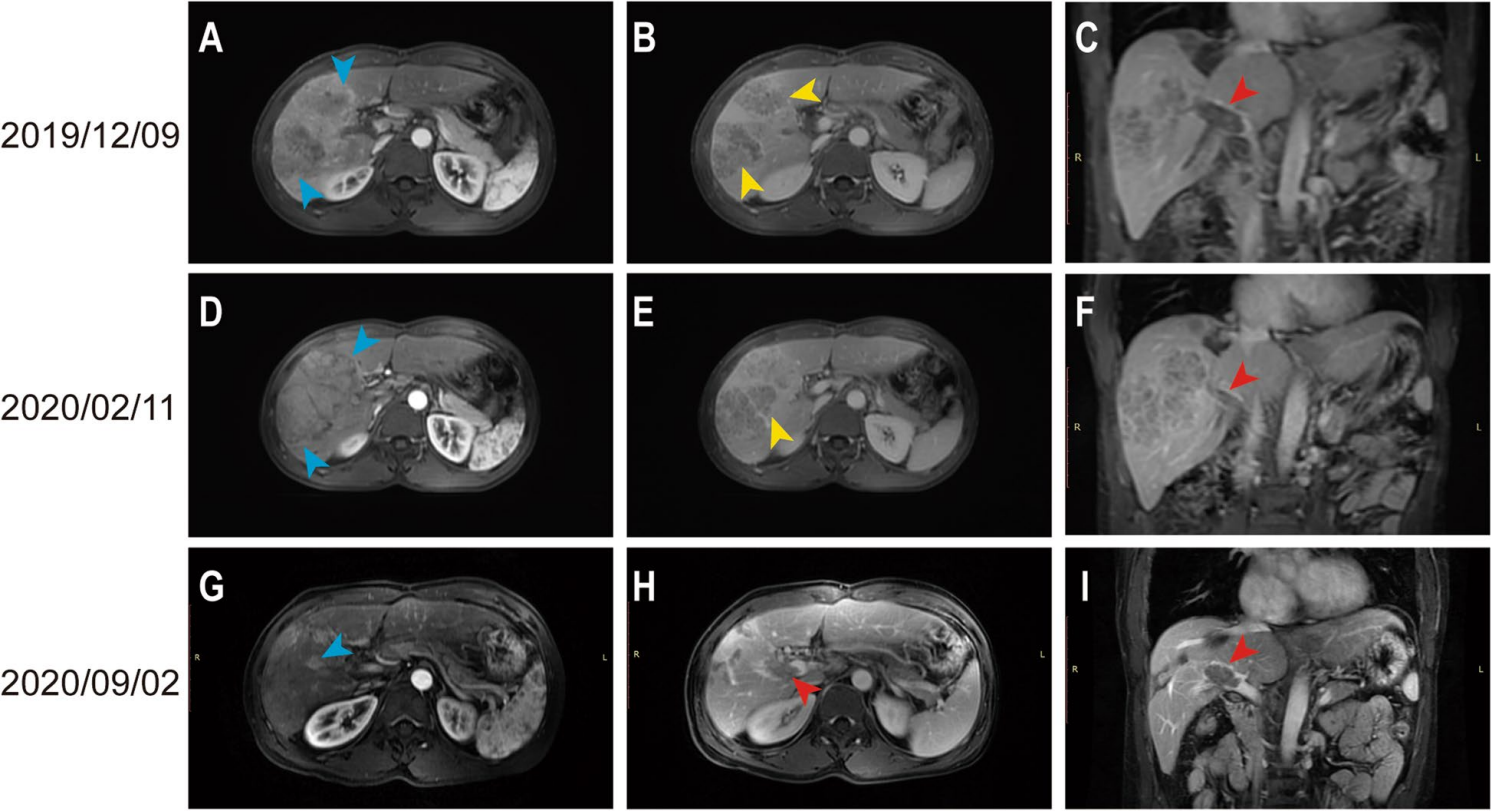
MRI examination of patient A during the combined treatment. A–C Before administering the combination therapy. D–F After 9 weeks of apatinib in combination with sintilimab therapy. G–I After 7 months of lenvatinib in combination with sintilimab therapy. The blue arrows indicate the position of lesions at the early arterial enhancement stage. The yellow arrows indicate the position of lesions at the portal vein stage. The red arrows indicate the position of PVTT
